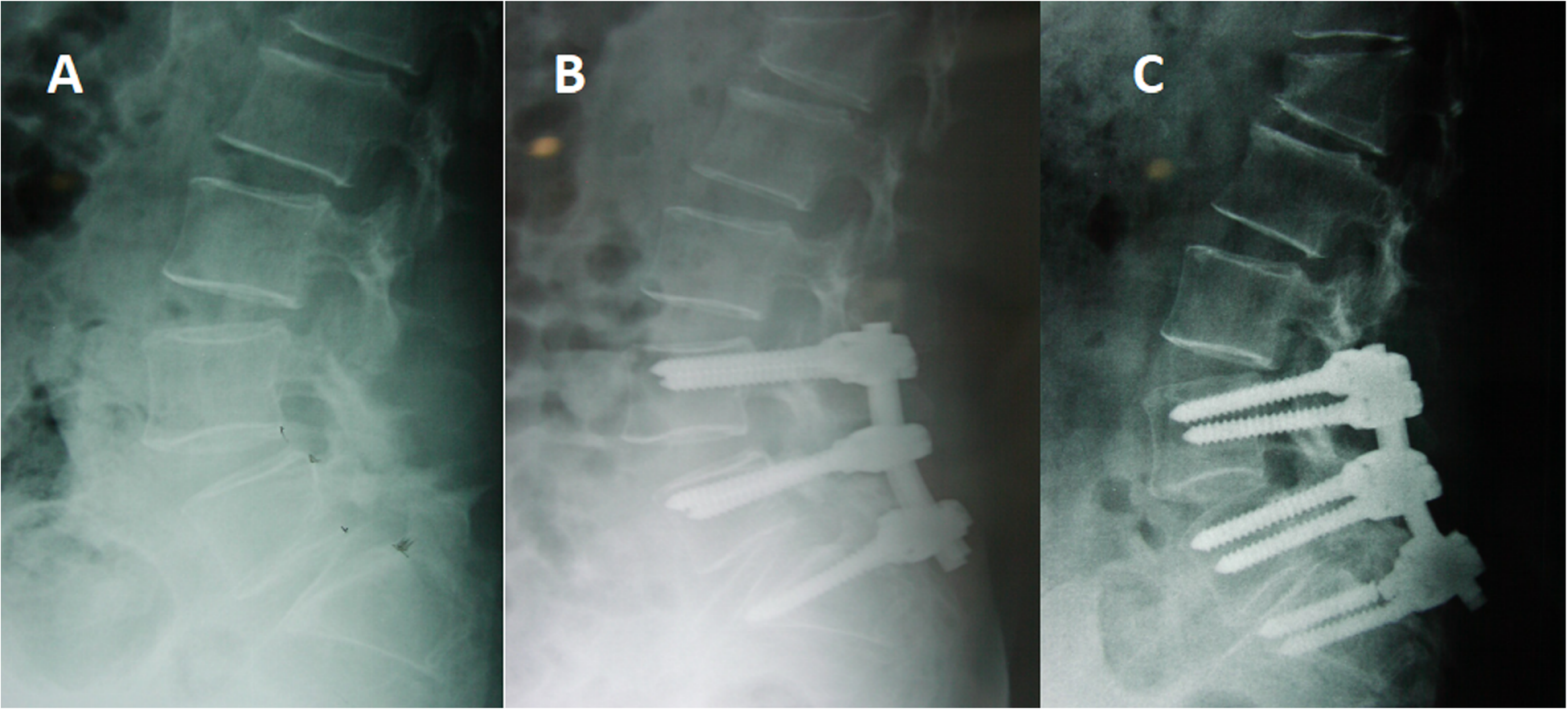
L4 DLS in a patient treated with L5 laminectomy and posterolateral fusion. (a, b) Postoperative X-ray was normal. c The X-ray showed screw displacement and fracture at the 36-month follow-up
